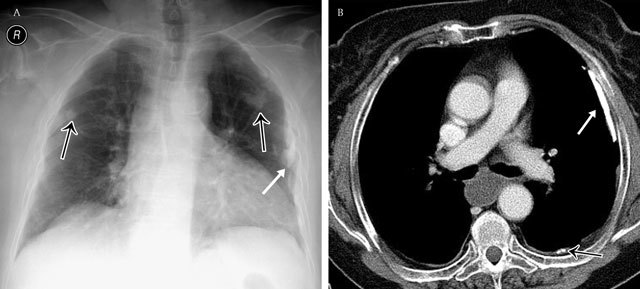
Asbest-related pleural plaques in a 59 year-old builder – Frontal chest radiograph (a) shows leaf-like or “geographic” opacities when the pleural plaques are viewed en face (black arrows). Tangentially viewed, pleural plaques are seen as focal areas of pleural thickening (white arrows). Corresponding axial CT image (b).
